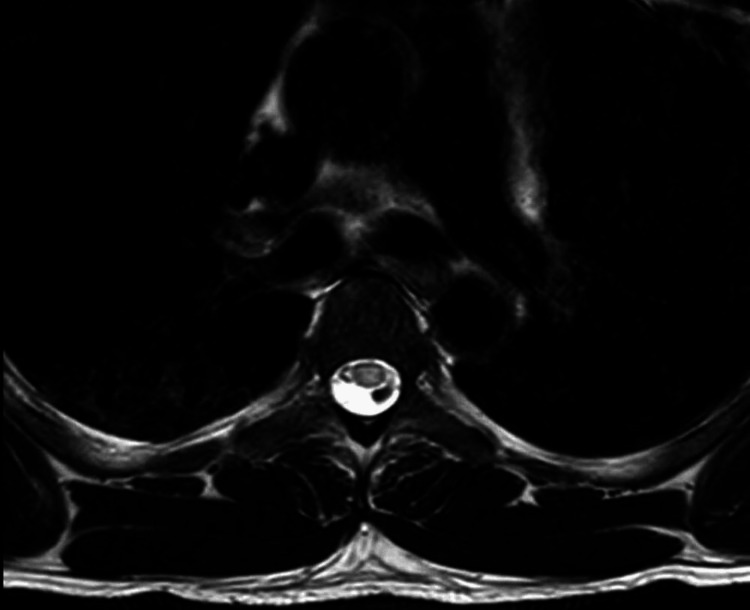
MRI Thoracic spine - Axial view showing no abnormal enhancement
